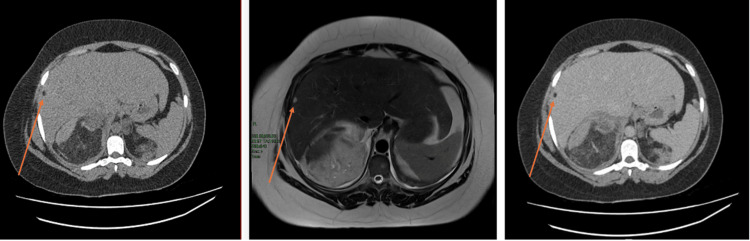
Axial views of hepatic AML in CT kidney triple phase (non-contrast phase), MRI abdomen T1-weighted and CT kidney triple phase (contrast-enhanced portal venous phase) The figure shows axial views with arrows highlighting the reported 8 mm focal hepatic angiomyolipoma within segment VIII of the liver. This is seen first across the non-contrast CT image (image on the left), with a corresponding lesion on the second T1-weighted MRI image (central). The final image is contrast-enhanced, and the lesion is again highlighted (image on the right). AML: angiomyolipoma
